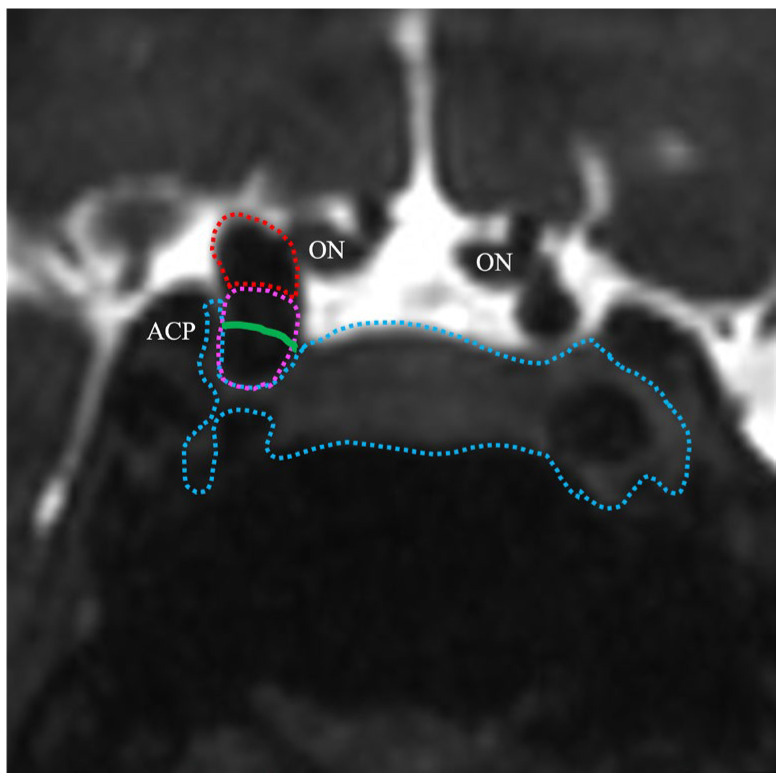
CISS MRI image of a representative discordant case classified as TS–ACP line–I but confirmed as DDR–S. CISS shows the parasellar anatomy: the ICA (red dotted outline), the aneurysm (pink dotted outline), and the cavernous sinus (blue dotted outline); the green line indicates the DDR. ON: optic nerve, ACP: anterior clinoid process, DDR: distal dural ring, CISS MRI: constructive interference in steady state magnetic resonance imaging, TS: tuberculum sellae.
